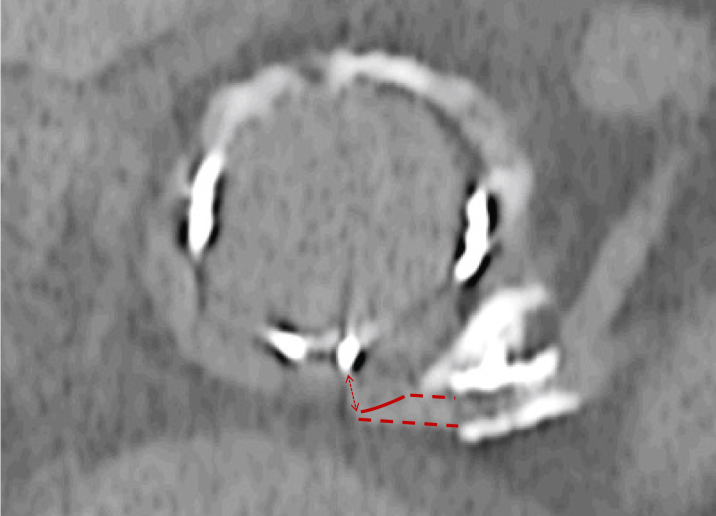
Computed Tomography Reconstruction Post Percutaneous Coronary Intervention CT reconstruction demonstrating the LMS stent following the first procedure, with red lines representing the distance to the left main ostium.
